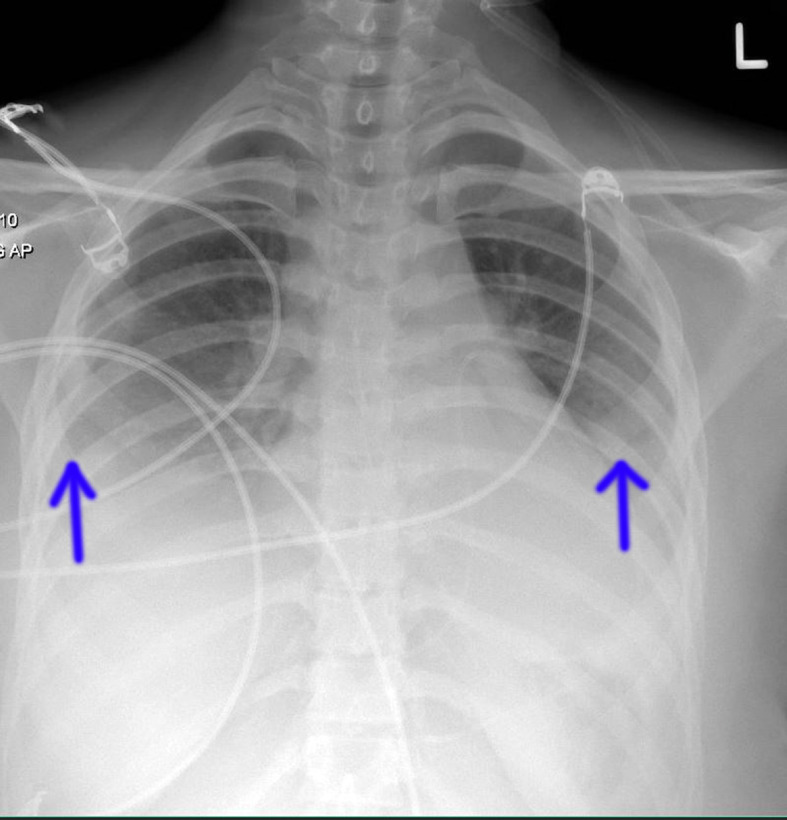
Chest X-ray at the time of initial presentation showing bilateral obliteration of the costophrenic angles, more pronounced on the right side, and haziness in both lower lung fields (blue arrows).
